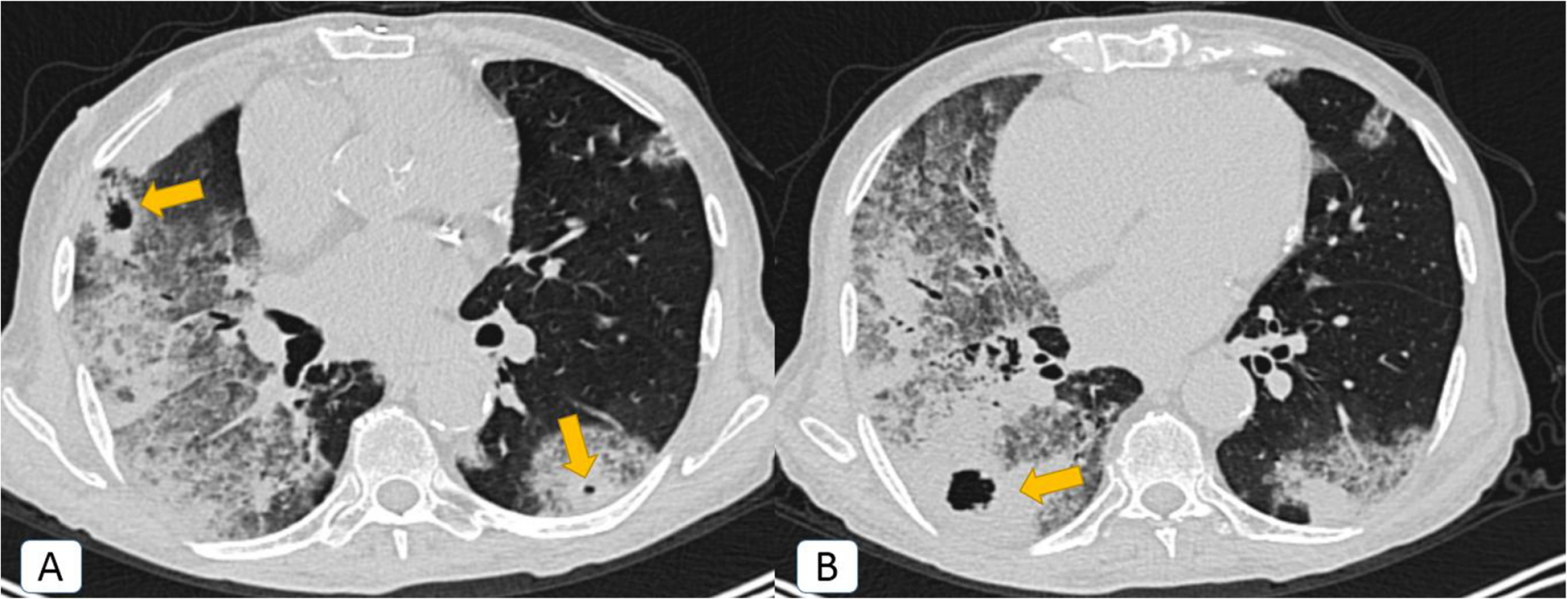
COVID-19 infection atypically presented by cavitation: a 62-year-old male COVID-19 patient. A, B Axial chest CT (lung window) showing bilateral widespread mixed ground glass and consolidative patches are noted (more pronounced on the right side). Secondary cavitary changes are noted (orange arrows)
